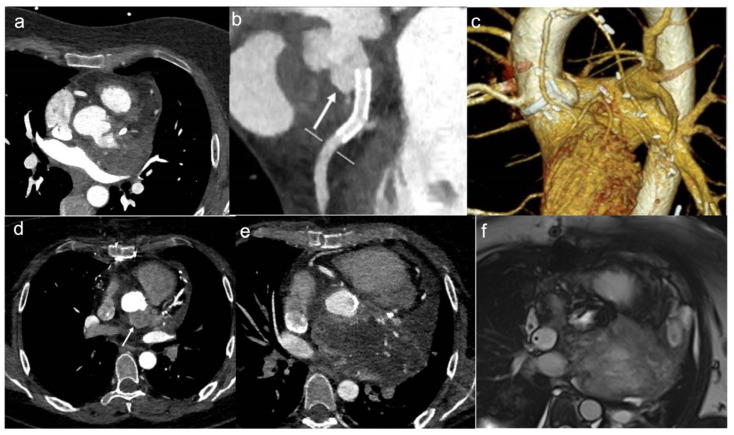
A 46-year-old woman with retrosternal pain during exertion and non -ST elevation myocardial infarction underwent coronarography. The interventional procedure showed stenosis of the common trunk treated with bifurcation stent implantation. Later, due to the unexplained pericardial effusion, the patient underwent coronary computed tomography angiography (CCTA) which revealed a voluminous pseudoaneurysm (arrow) of the left coronary sinus (a,b). Cardiac surgery was performed to exclude pseudoaneurysm and to perform a by-pass (c). After the cardiac surgery, the patient performed a CCTA that showed the exclusion of the pesudoaneurysm (d). Four months later, when the patient was back for a follow-up, CT angiography showed a mass with peripheral enhancement, central hypodensity due to necrosis, and central neoangiogenesis (e). Finally, the patient underwent CMR that confirmed the presence of inhomogeneous mass invading the surrounding structures (f) and neoplasia was suspected. Lastly, the ultrasound-guided transesophageal biopsy has shown the presence of fused and pleomorphic malignant cells with leiomuscular immunophenotype (actin 1A4 +) and the diagnosis of leiomyosarcoma was made.
